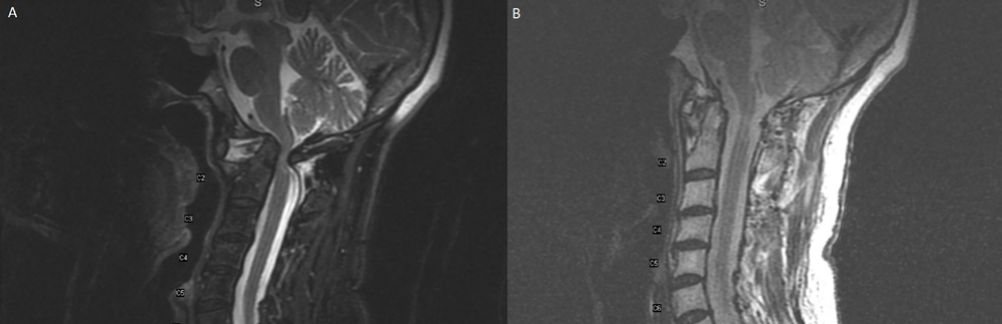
A: Preoperative T2-weighted sagittal MR image demonstrating severe craniocervical junction canal stenosis secondary to basilar invagination from C1–2 joint subluxation. B: Postoperative T2-weighted sagittal MR image demonstrating dramatic decompression of craniocervical junction and C1–2 reduction supported by C1–3 instrumented fixation.
